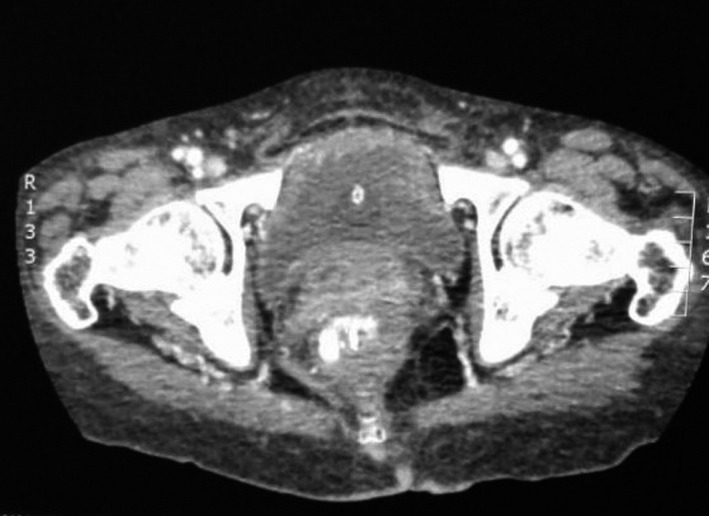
CT scan images showing resolution of emphysematous cystitis after 10 days of antibiotics
